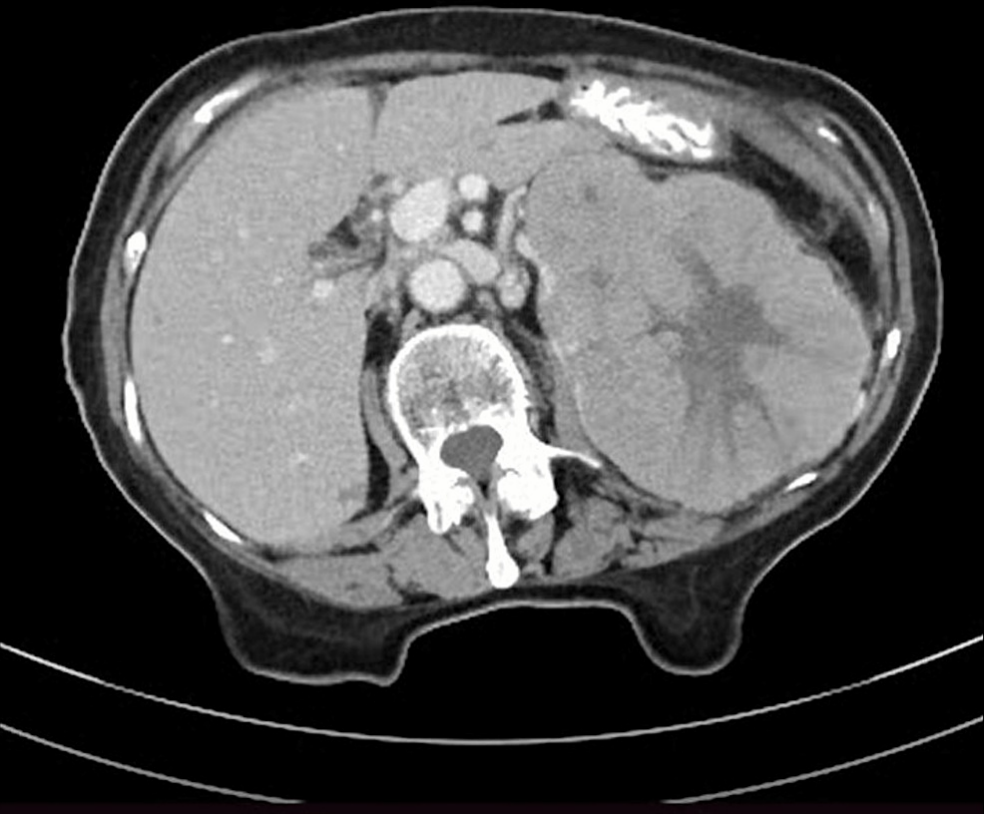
CECT of the abdomen demonstrating a large left renal mass, featuring central non-enhancing areas indicative of necrosis or scarring. CECT, contrast-enhanced computed tomography
